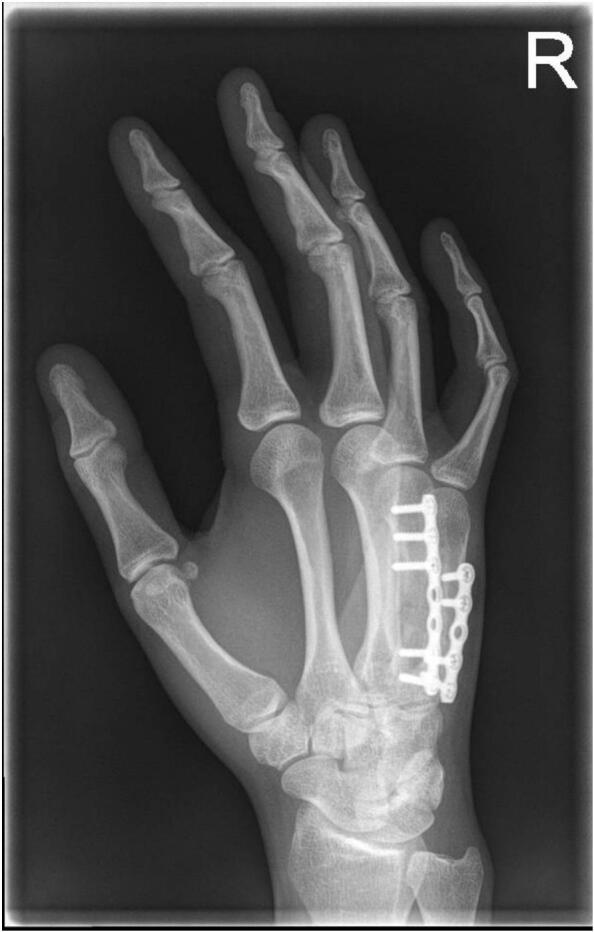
Radiograph at sixteen weeks post-iliac cancellous bone grafting (second phase of the Masquelet procedure) and plate osteosynthesis. Nearly complete consolidation of metacarpal IV was achieved.
